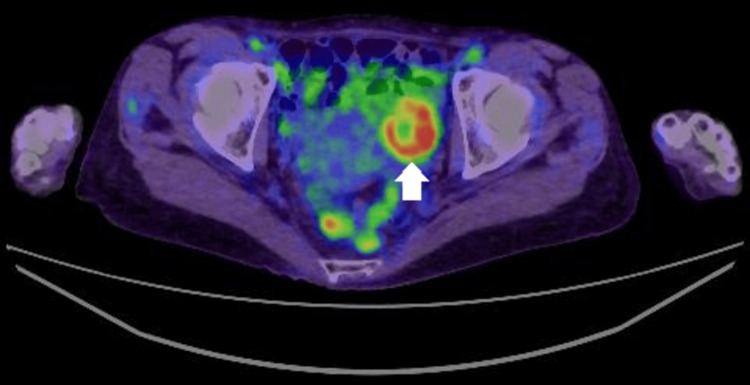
Pelvic positron emission-CT imaging Uptake in the solid component of the left ovarian tumor was observed (arrow).
